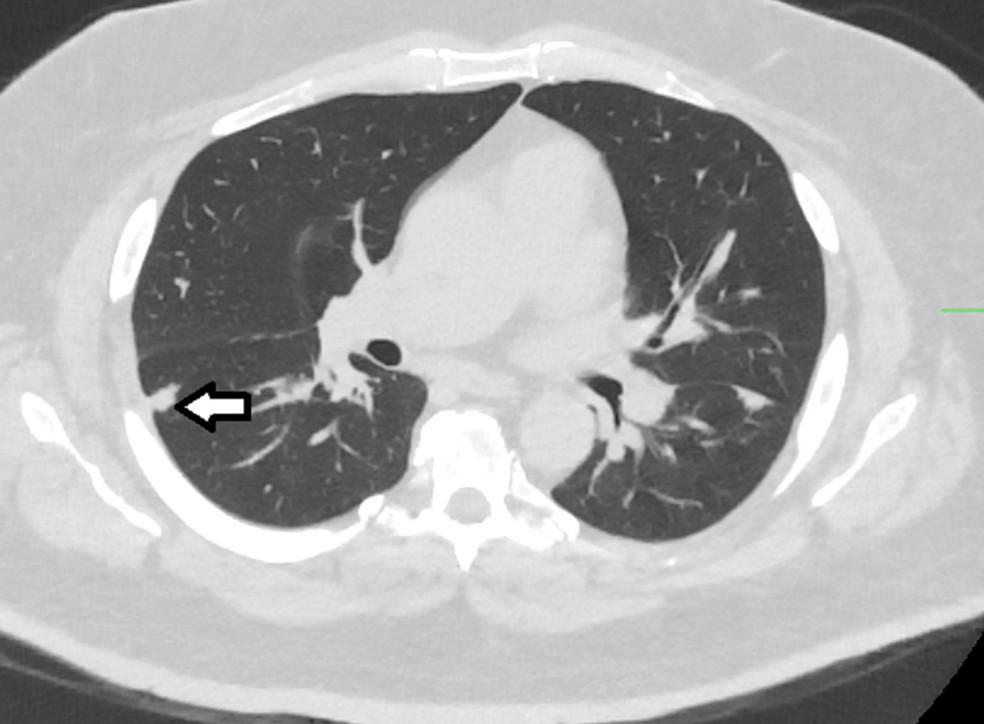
CT chest (lung window) (axial view). Multiple subcentimeter pulmonary nodules, Right lower lobe nodule (arrow).
